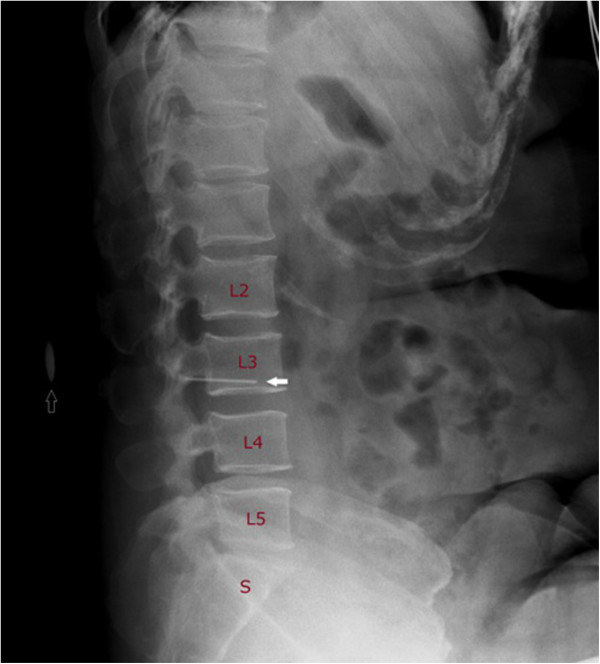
A lumbar spine X-ray in the hyperflexion position. The vertebrae from L2 to S are indicated. The solid arrow shows the radio-opaque marker where the highest point of the iliac crests was identified by palpation. The hollow arrow represents the spinous interspace identified by an anesthesiologist (the L2-L3 spinous process interspace in this film).
